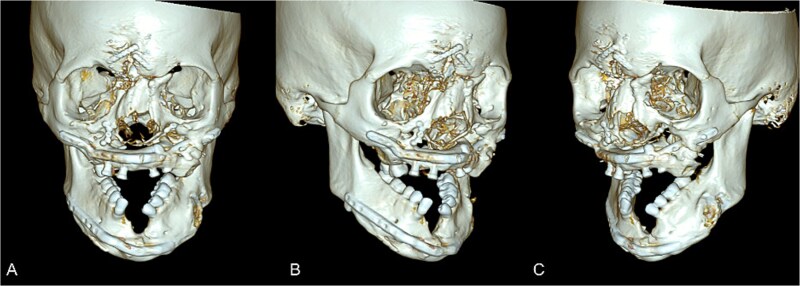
Postoperative maxillofacial CT with 3-D volume following revision surgery. The reconstructed left midface/mandible with is shown after removal of the osseous bridge. (A) Frontal view. (B) Right anterior view. (C) Left anterior view.
